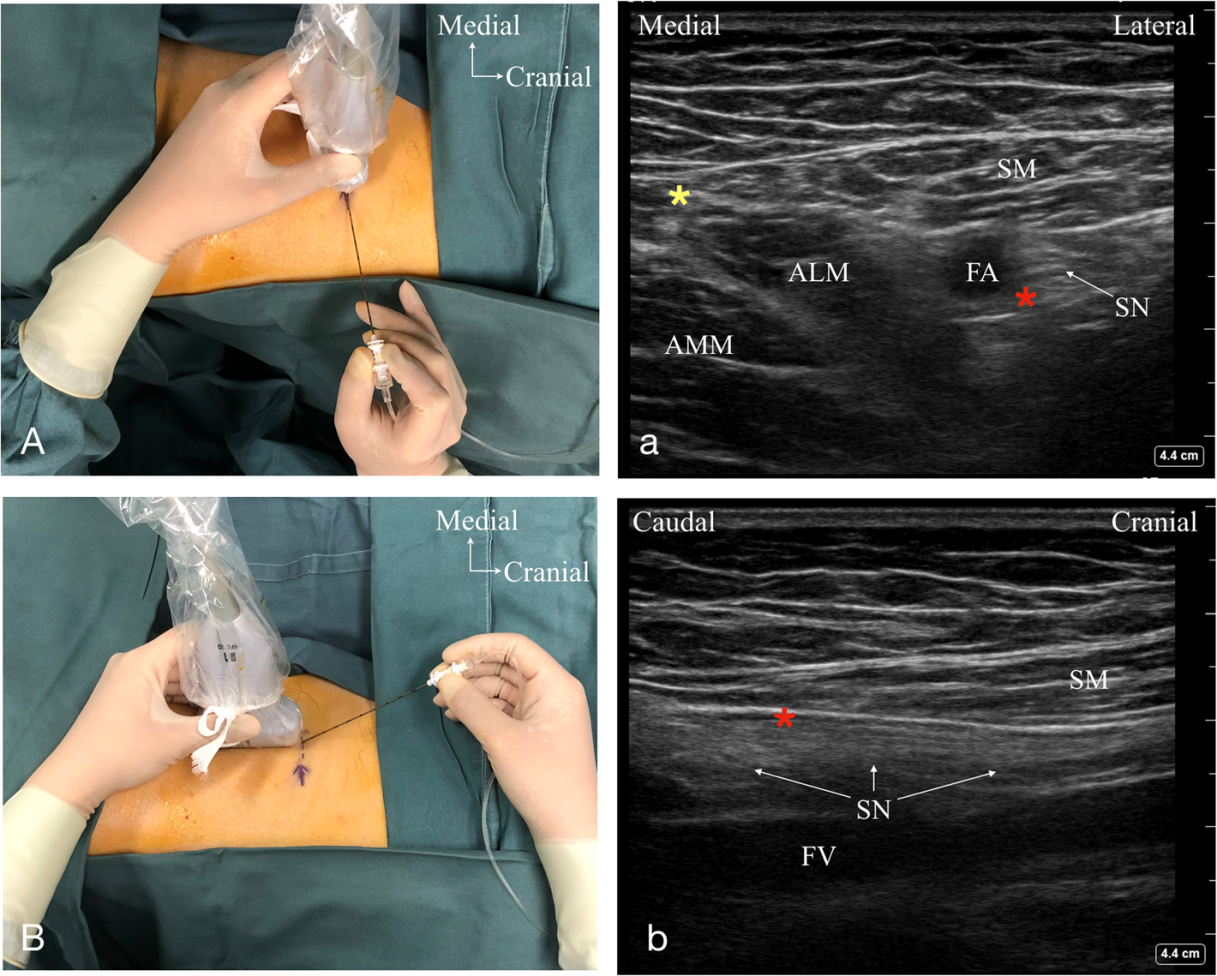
Ultrasound-guided proximal end adductor canal block (ACB) (A/a) and middle ACB (B/b) techniques. (A) Ultrasound probe position of short-axis scanning at the proximal end of the AC and needle orientation for proximal end ACB. (a) Short-axis ultrasound scan image at the proximal end of the AC. (B) Ultrasound probe position of long-axis scanning with the cranial end of the probe aligned with the proximal end of the AC and needle orientation for middle ACB. (b) Long-axis ultrasound scan image with the cranial end of the probe aligned with the proximal end of the AC (at the cranial side in the image). The purple arrow indicates the skin mark of the puncture point for proximal end ACB; the purple dotted line indicates the skin mark of the proximal end of the AC; the red asterisk indicates the endpoint target for the needle tip; the yellow asterisk indicates the alignment of the medial borders of the SM and ALM. ALM, adductor longus muscle; AMM, adductor magnus muscle; FA, femoral artery; FV, femoral venous; SM, sartorius muscle
